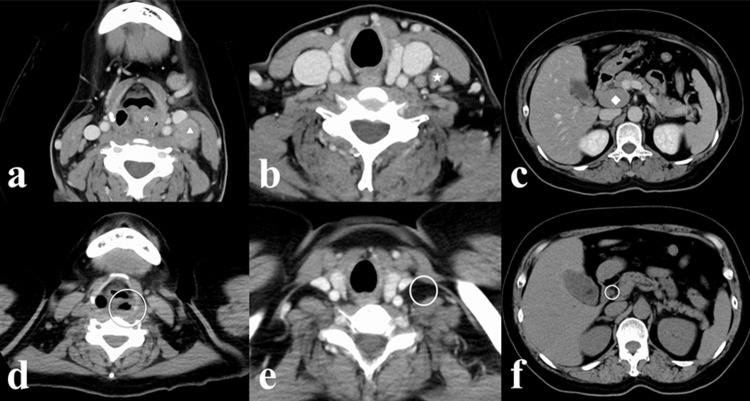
Findings on computed tomography (a-c) A contrast-enhanced scan obtained on initial presentation shows a 15×11×22 mm iso-density mass centered on the left piriform sinus extending to the arytenoid and post-cricoid area and bulged toward the laryngeal cavity (*). Lymphadenopathy was observed in the left superior (△), middle (☆), and inferior internal jugular nodes of the cervical region and in the hepatoduodenal mesentery (◇). (d-f) After one course of chemotherapy using the mLSG15 protocol, there was a marked reduction in the size of the lesion.
